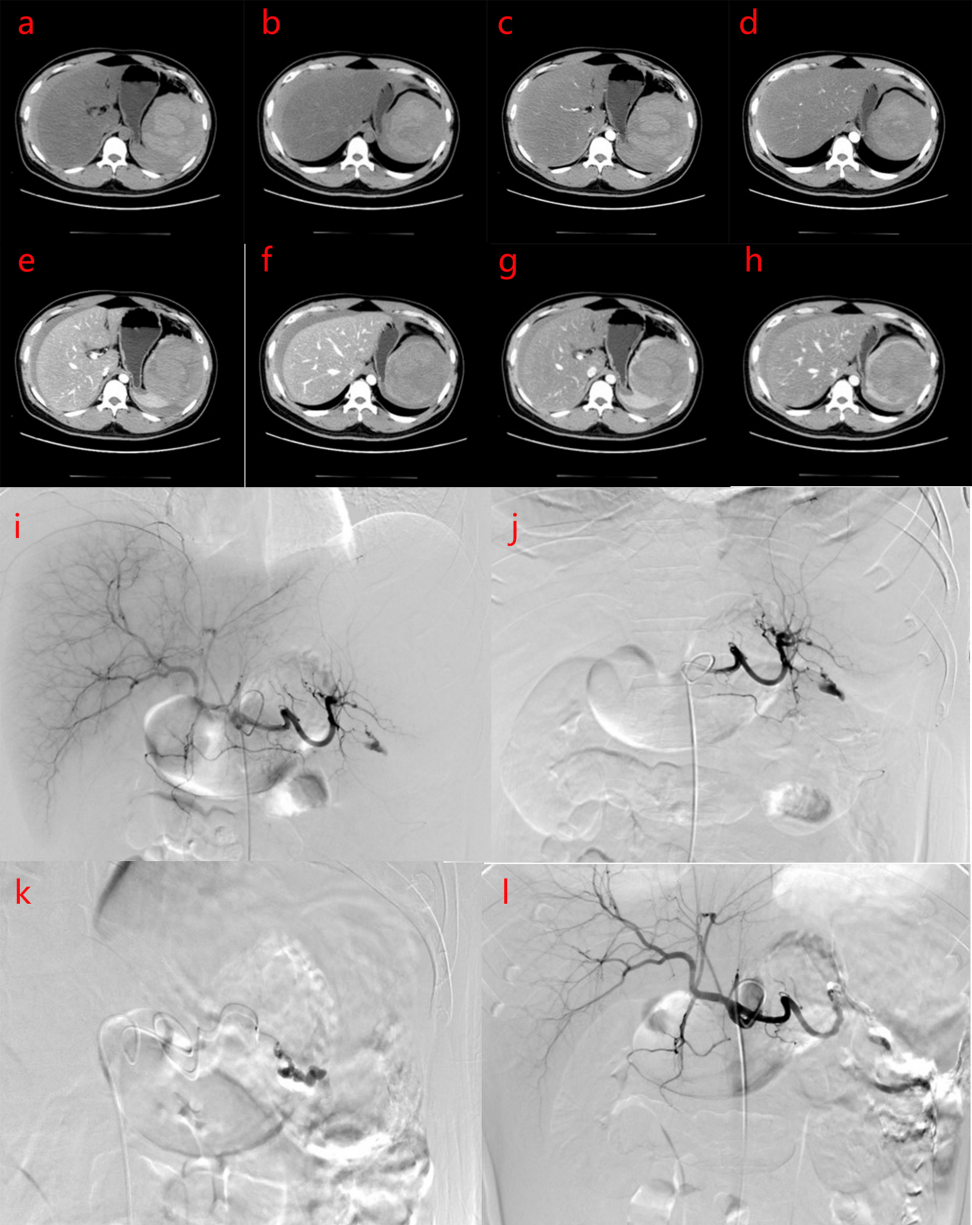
(a–h) Plain computed tomography (CT) scan and enhancement of the upper abdomen CT before intervention shows that the density of liver parenchyma is significantly reduced, and the shadow of intrahepatic vessels shows relative density on plain CT scan. The spleen is enlarged, and there is a mixed density shadow in the spleen with a size of about 10.0 × 8.8 × 12.5 cm. The plain CT value is about 11–67 HU, the boundary is unclear, and the enhanced CT shows partly obvious progressive lesion enhancement. Patchy and slightly high-density shadows were observed around the spleen, liver, and abdominal space, and the CT value is about 44–71 HU. The clinical impression is neoplastic lesions with rupture and hemorrhage, which may be vascular tumors. Intraperitoneal accumulation of blood and fluid. Severe fatty liver. (a,b) PLAIN CT scans; (c,d) Arterial phase of CT; (e,f) Portal veinphase of CT; (g,h) Delayphase of CT. (i–l) Digital subtraction angiography (DSA) and interventional embolization of the spleen. DSA showed splenic artery rupture and bleeding, abdominal fluid accumulation.
